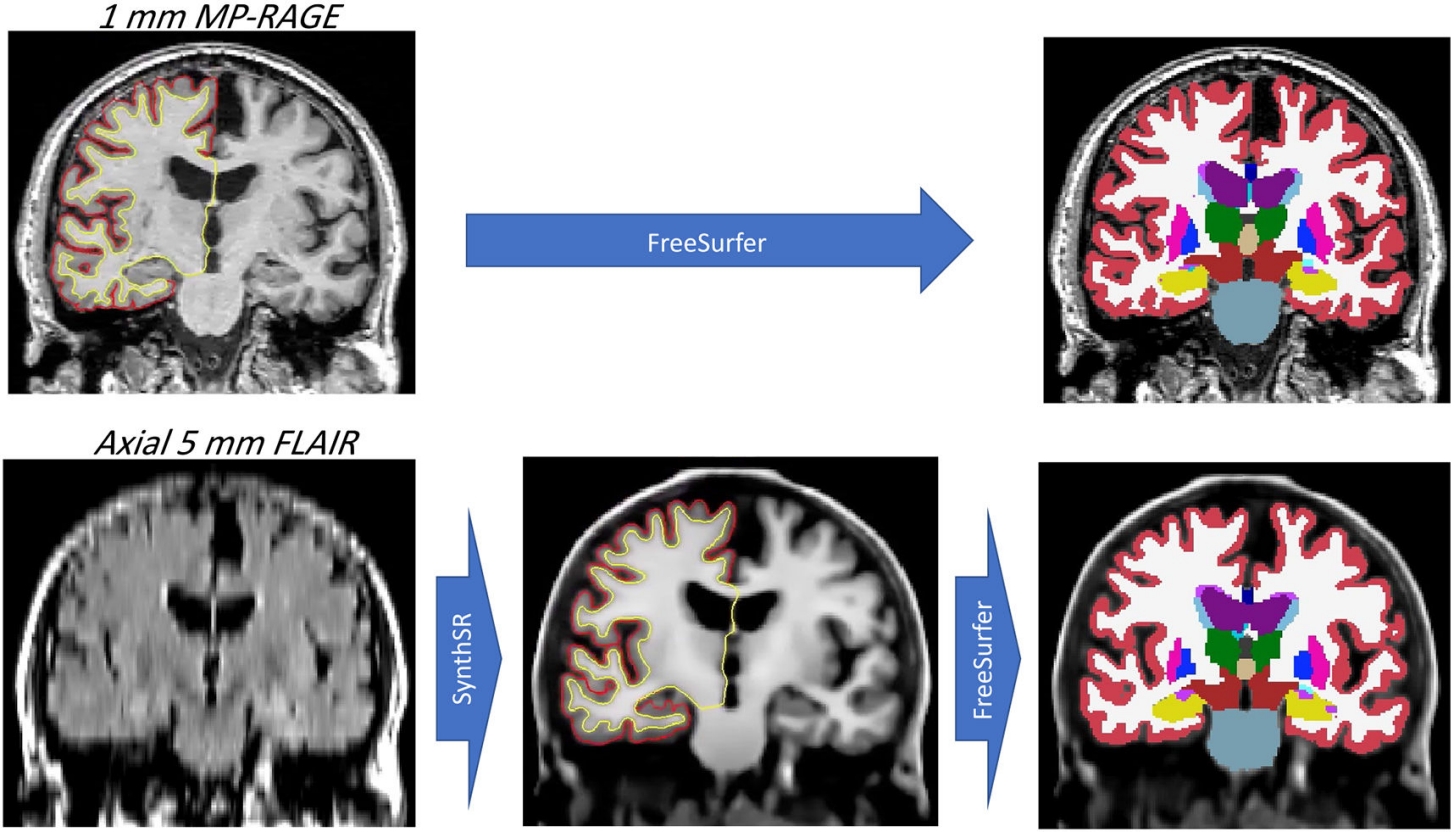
Left column: coronal plane of an MP-RAGE scan (top, slice thickness: 1 mm)) and corresponding coronal plane of an axial FLAIR scan (bottom, slice thickness: 5 mm) from the ADNI dataset (adni-info.org). Middle column: synthetic 1 mm MP-RAGE volume produced by Dr. Iglesias’s tool. Right column: automated segmentation of the original and synthetic MP-RAGE volumes produced by FreeSurfer (Fischl et al., 2002)
